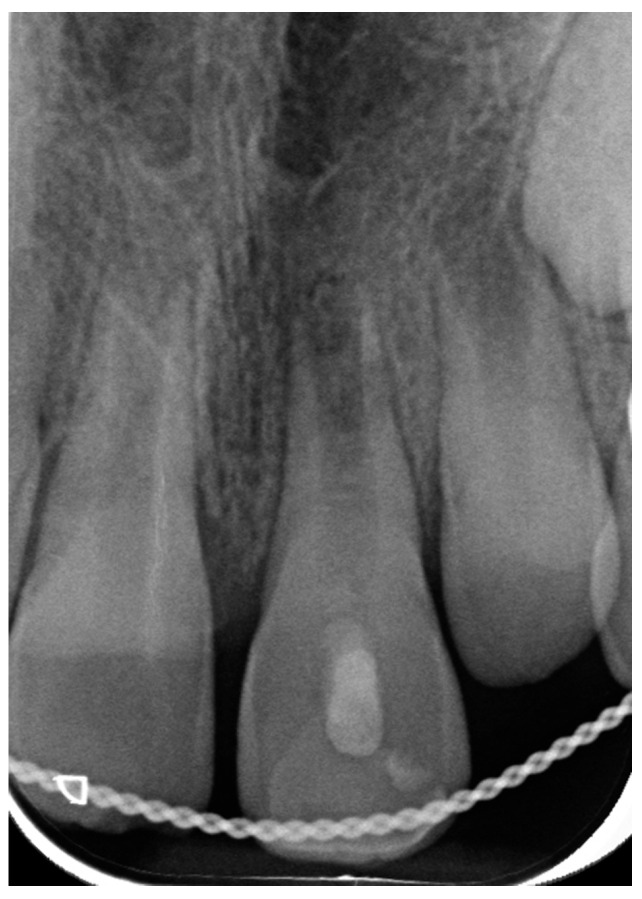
Case 1, one month following avulsion. Radiographs exhibited initial evidence of inflammatory resorption. Clinically, a metal sound in percussion with no detectable mobility suggested the tooth was ankylotic.
